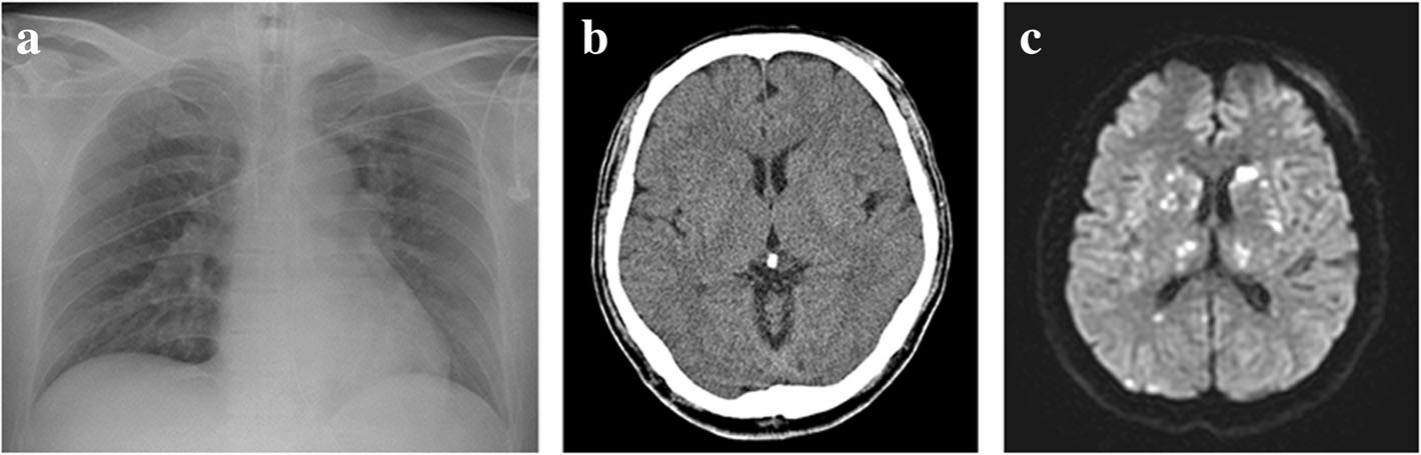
a A chest X-ray of a clear lung field. b A brain CT showing no intracranial hemorrhage. c A brain MRI showing multiple nodular or punctate hyperintensity lesions on diffusion-weighted (DW) images of the bilateral thalami, basal ganglia, cerebellar, and cerebral hemispheres
